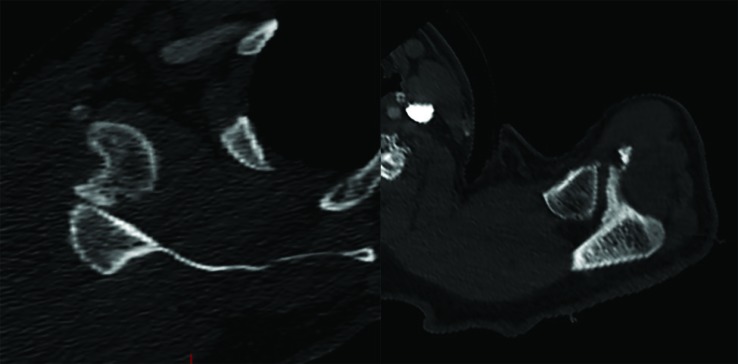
Computerized tomography (CT) scan reconstruction images post-initial reduction. Hill-Sachs Impaction and Bankart fracture seen on the right shoulder (Left image). Avulsion fracture of left acromion (Right image)
